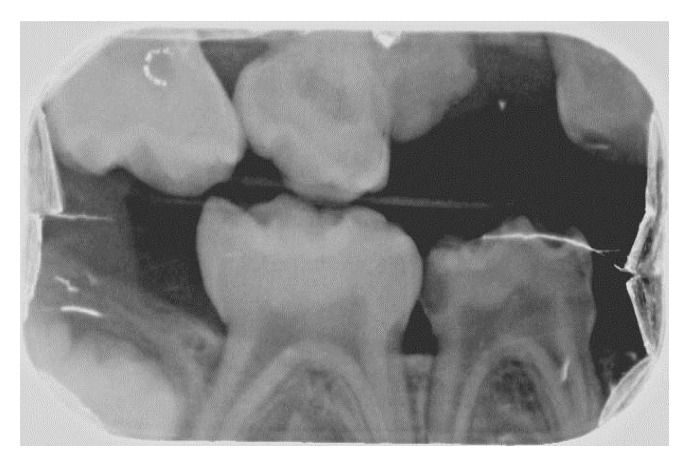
The right bitewing radiograph shows a suspicious area mesial to the maxillary right first primary molar. Caries are noted on the distal surface of the mandibular right first primary molar.
